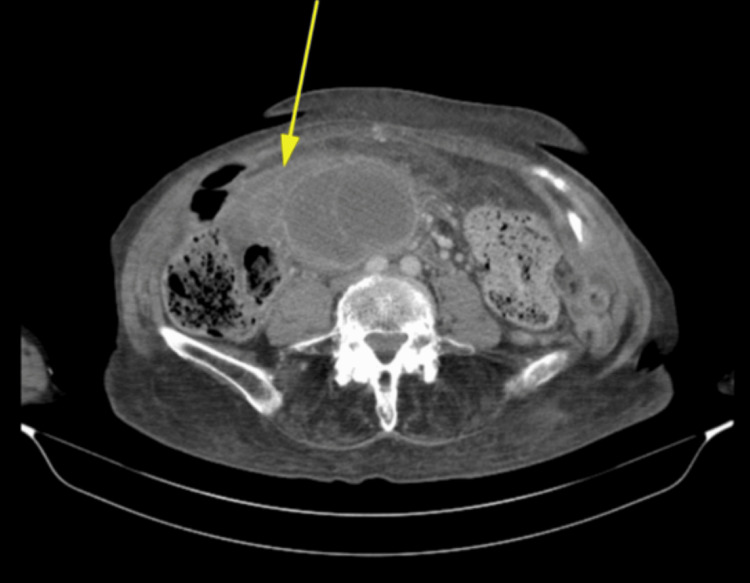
Axial CT of the Abdomen Showing a Large Epigastric Mass Displacing Adjacent Bowel Loops Axial CT image of the abdomen and pelvis demonstrating a large, well-defined, heterogeneous mass in the epigastric region, extending toward the right upper quadrant (arrow). The mass displaces adjacent bowel loops and was initially suspicious for intra-abdominal malignancy.
